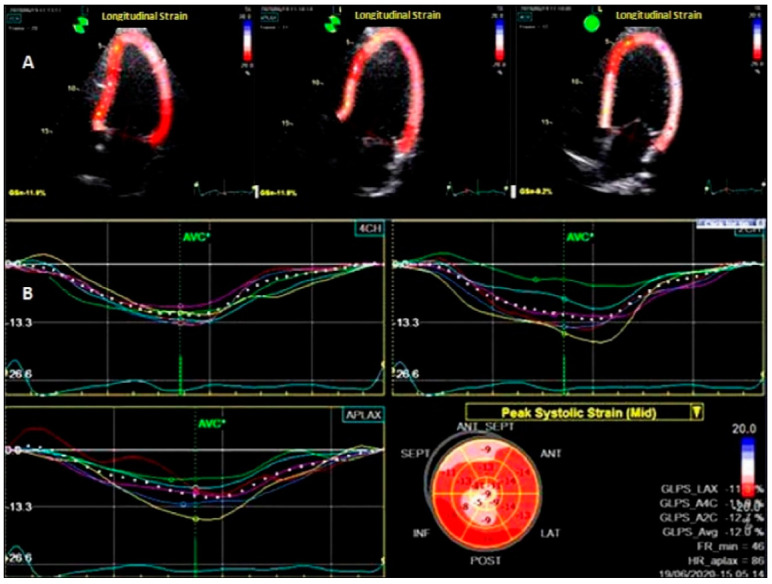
Two–dimensional speckle–tracking echocardiography at the level of the left ventricle. (A) Longitudinal analysis of deformation in apical 2-, 3-, and 4 chamber views; (B) bull’s eye map.
